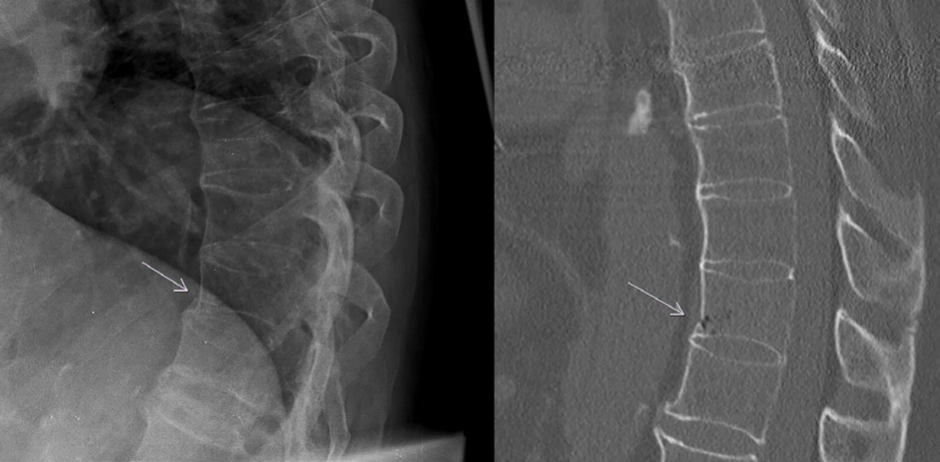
Fracture in a 70-year-old men with known ankylosing spondylitis. Radiography (on the left) shows only a very subtle kinking at the ventral cortex of TH 11. The CT-scan (on the right) confirms this finding
